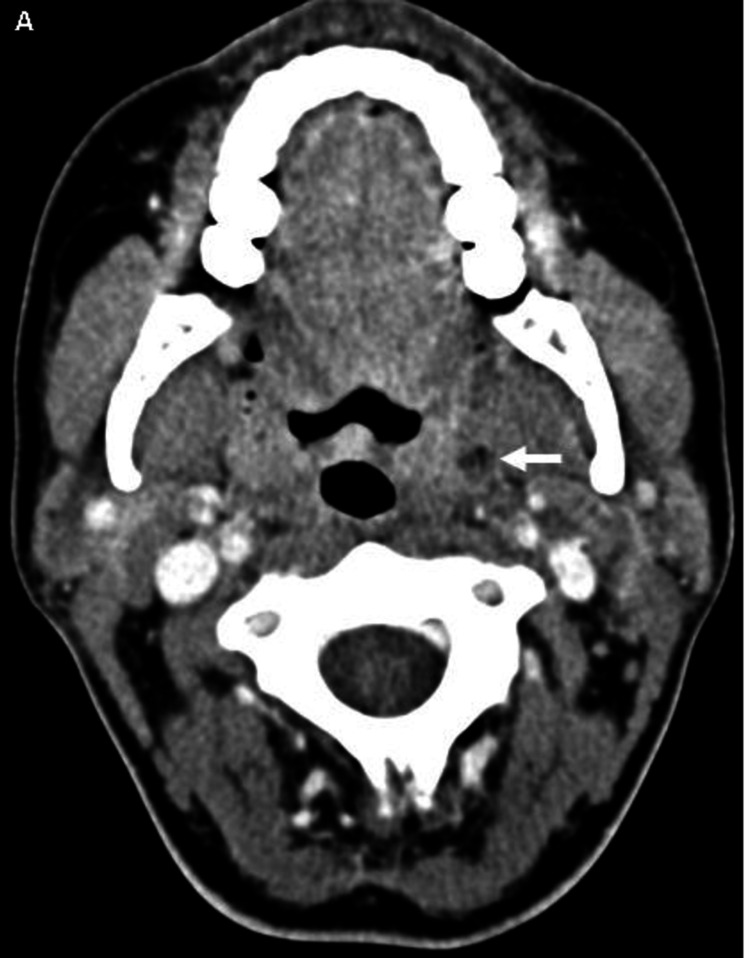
CT axial with IV contrast On axial contrast-enhanced CT, the left palatine tonsil demonstrates a small focus of low attenuation with an internal gas locule compatible with a small peritonsillar abscess (white arrowhead) CT: computed tomography; IV: intravenous
